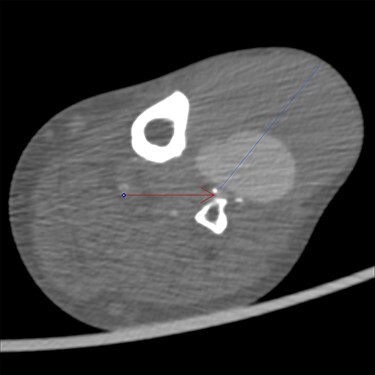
CTA showing the pseudoaneurysm at 75 mm (blue line) with both thrombus and contrast, and the patent anterior tibial artery (red arrow). Original magnification: 2000 × 2000 pixels.
